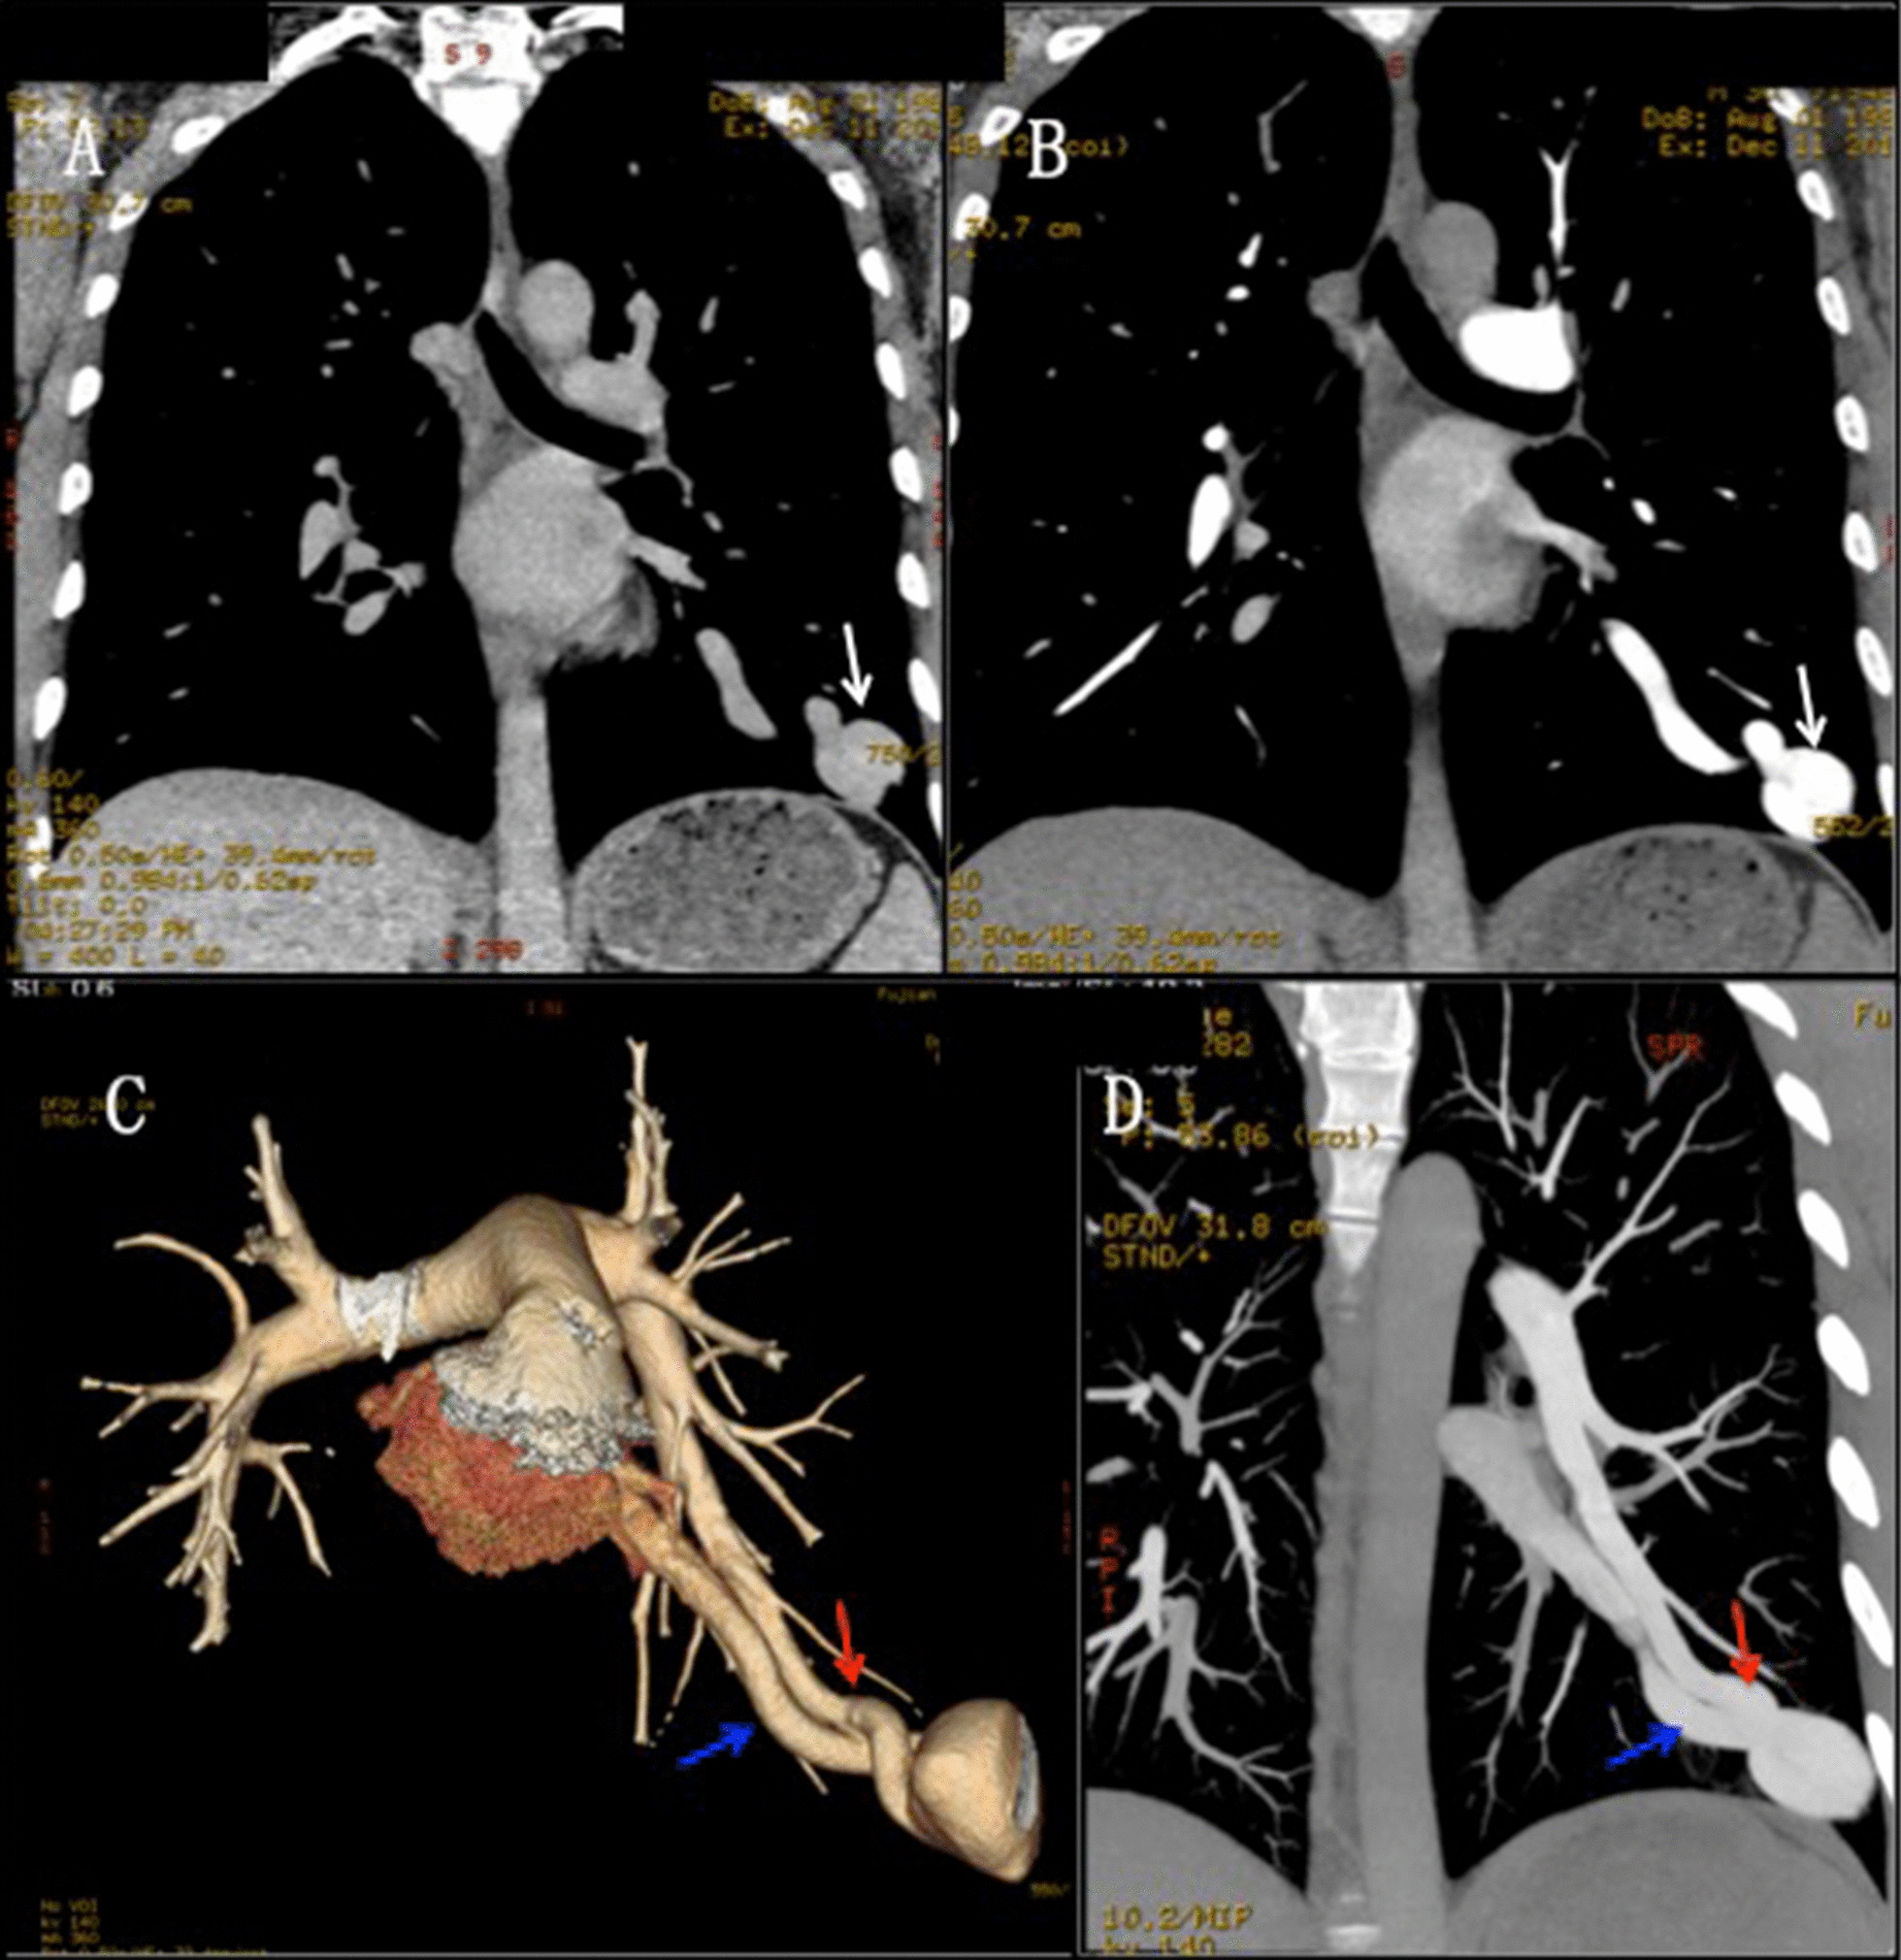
a CT scan shows a massive lesion in the left lower lobe (arrow). b Enhanced CT shows a massive lesion with suspicion of a vascular malformation (arrow). c, d CTA and 3-dimensional reconstruction confirm the presence of one PAVM between the lower left pulmonary artery and the pulmonary vein in the left lower lung (red arrow and blue arrow)
